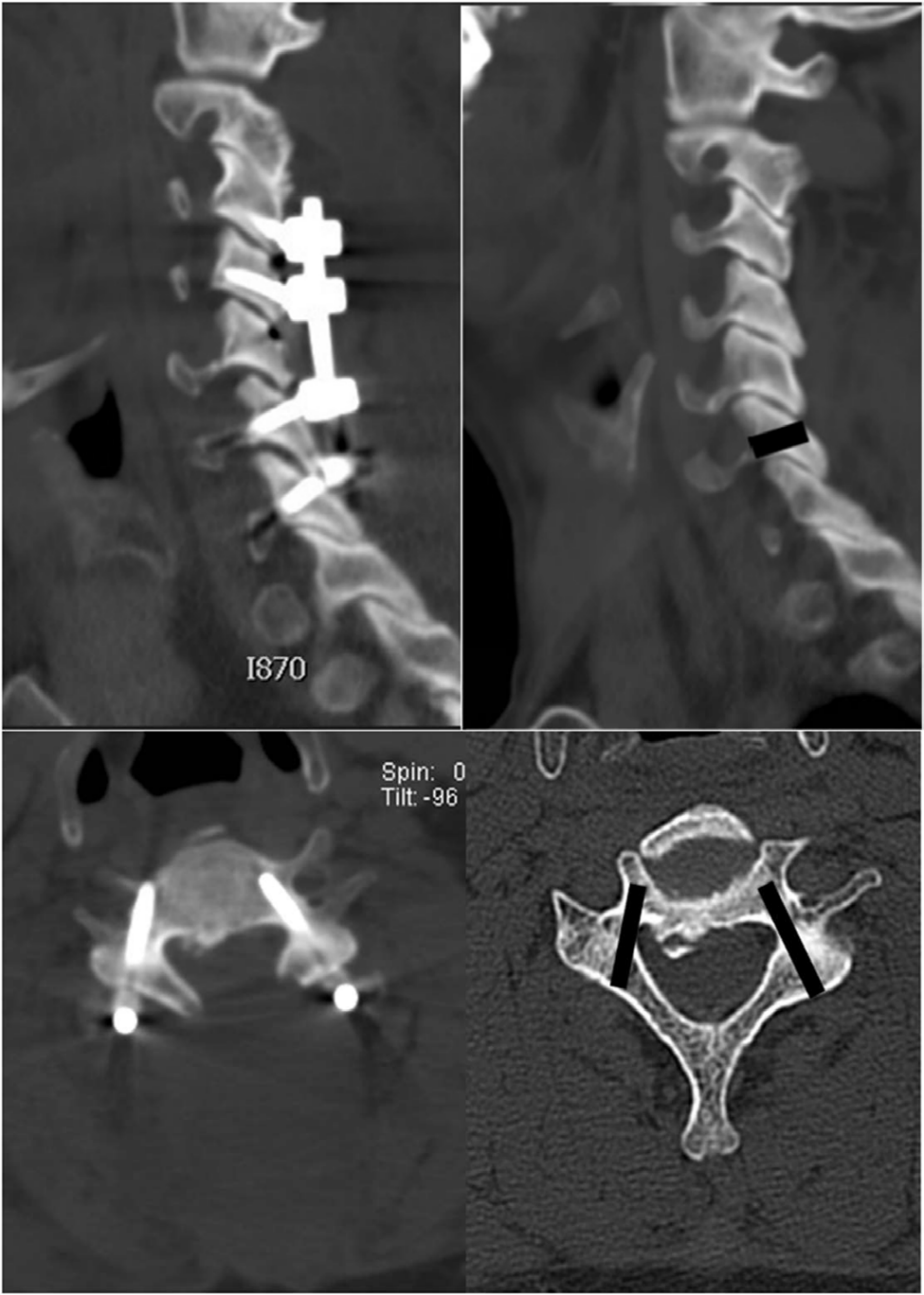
Extraction of cylindrical area of screw trajectory, screw position is confirmed by the postoperative CT
